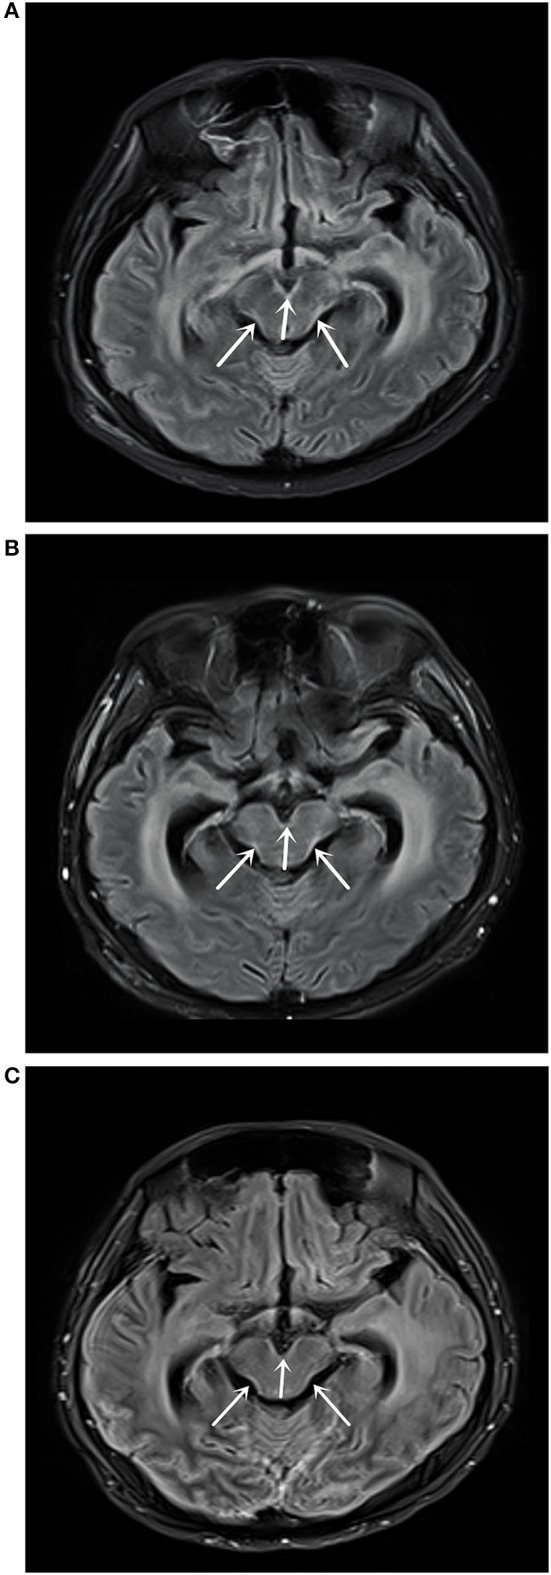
(A) Magnetic resonance imaging (MRI) T2 fluid-attenuated inversion recovery (FLAIR) enhanced scan on May 10, 2018 showed abnormally high signal shadows in the medulla oblongata, pons, and ventral and dorsolateral line of the midbrain, suggesting soft leptomeningeal metastasis. (B) MRI T2 FLAIR enhanced scan on July 31, 2018 showed medulla oblongata, pons, midbrain ventral, and dorsolateral line abnormally high signal shadows, with no significant changes compared with the MRI results on May 10, 2018. (C) MRI T2 FLAIR enhanced scan on September 18, 2019 showed medulla oblongata, pons, midbrain ventral, and dorsal lateral abnormally high signal shadows.
